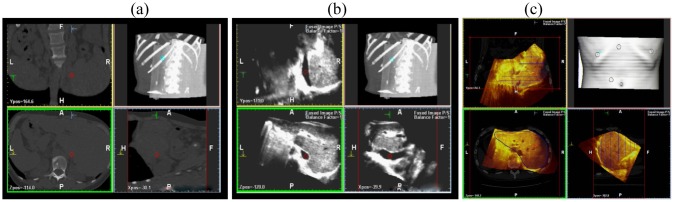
3-D reconstruction of bidimensional spiral CT sequences (a); bidimensional US sequences (b); the fusion technique allows to superimpose the two volumes, showing a perfect overlapping (c).
